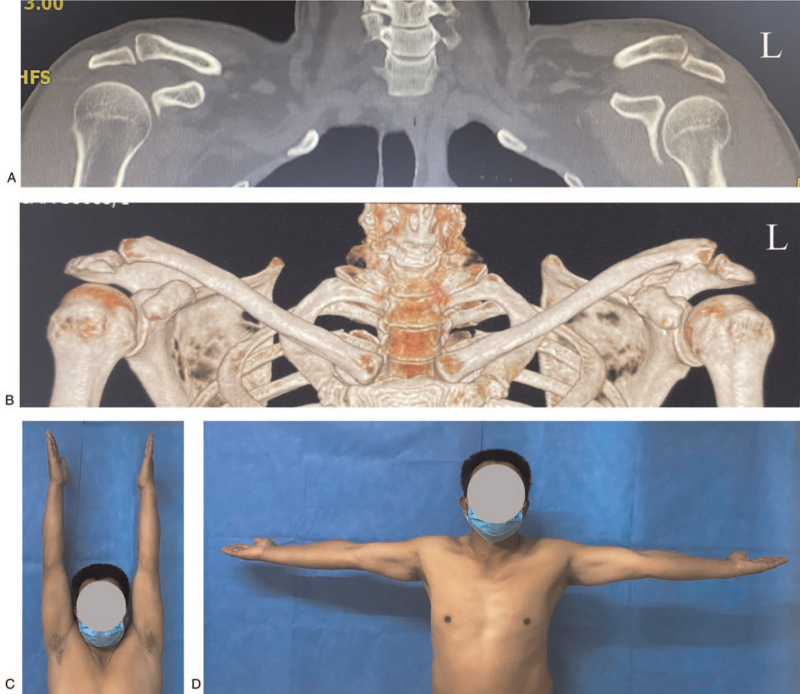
Two years after surgery. A. Coronal CT. B. Three-dimensional CT. C. Vertical flexion. D. Abduction. CT = computed tomography.
